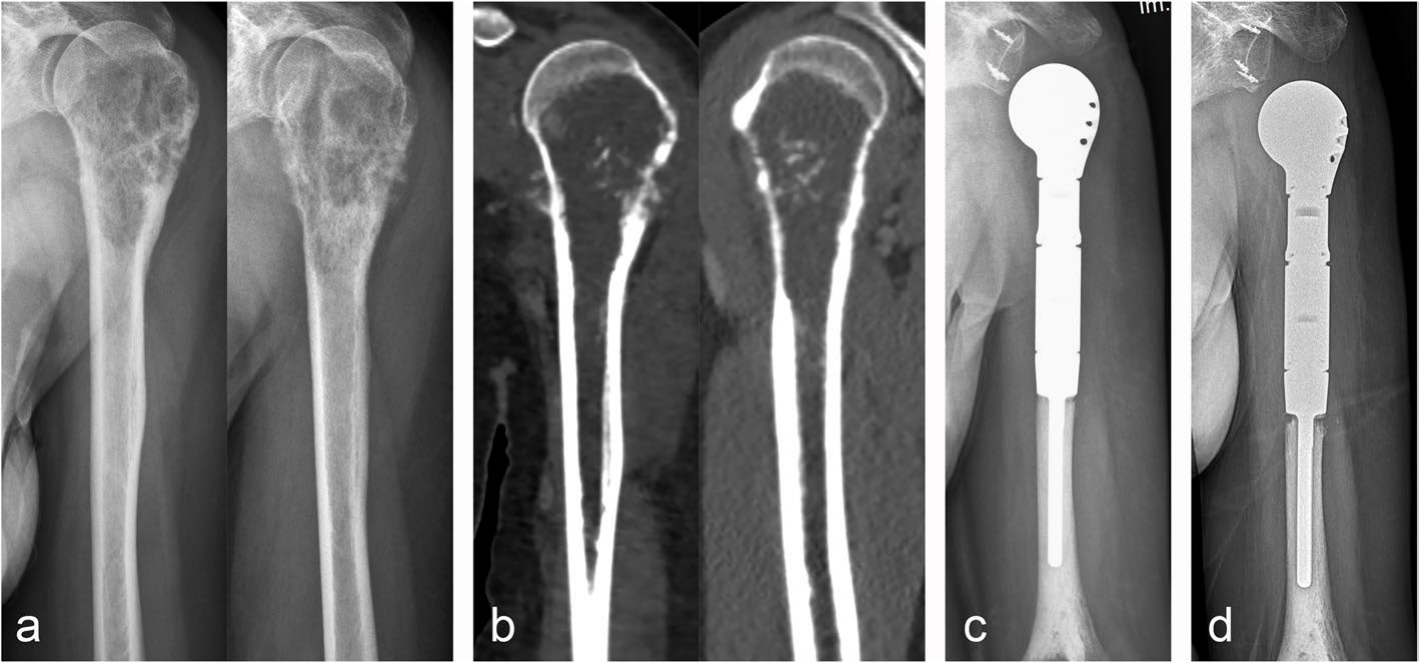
En bloc resection of osteosarcoma and reconstruction of tumor-type hemi-shoulder replacement. a Preoperative anteroposterior and lateral X-rays. b Coronal and sagittal CT scans showed extensive bone destruction with periosteal reaction. c Anteroposterior X-ray three months after surgery showed that the prosthesis was firmly fixed and the joint was stable. d The slightly downward displacement of the prosthesis was found at 1 year postoperatively
